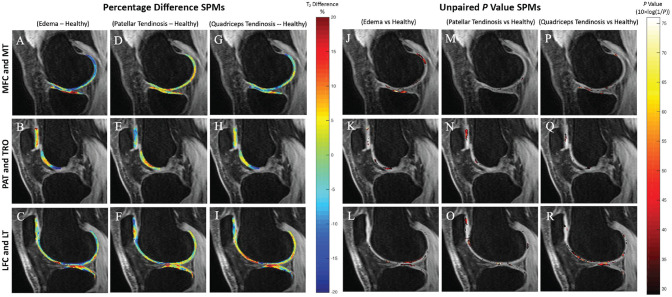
(A-R) Average cross-sectional T_2_ SPMs of all knees overlaid onto registered image. Percentage difference SPMs (A-I) and paired *P* value with a threshold set at *P* ≤ .05 (J-R) show regions of significant differences between knees with MRI-graded knee abnormalities (edema, patellar tendinosis, quadriceps tendinosis) versus healthy knees. In the percentage difference SPMs, red regions indicate higher T_2_ times in knees with an abnormality compared with healthy knees, while blue regions indicate lower T_2_ times. *P* values are represented as 10 × log(1/*P*). Analysis was adjusted for age, sex, BMI, and site of image acquisition. BMI, body mass index; LFC, lateral femoral condyle; LT, lateral tibia; MFC, medial femoral condyle; MRI, magnetic resonance imaging; MT, medial tibia; PAT, patella; SPM, statistical parametric map; TRO, trochlea.
